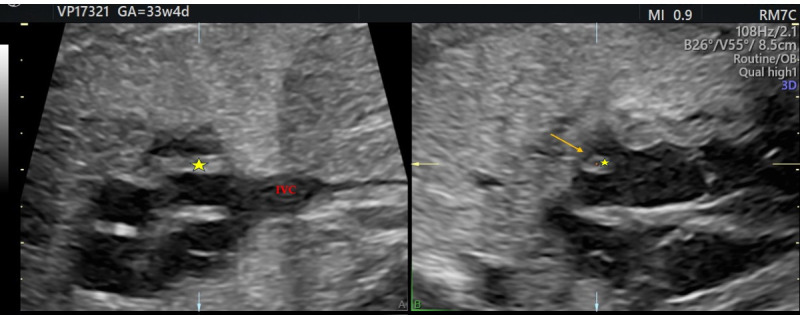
(A,B) Hypertrophic crista terminalis, ultrasound at 33 weeks + 4 days: a hyperechoic, round mass was seen in the right atrium (yellow arrow), which on 3D reconstruction appeared as a membranous structure traversing the atrial cavity (star). IVC: inferior vena cava.
